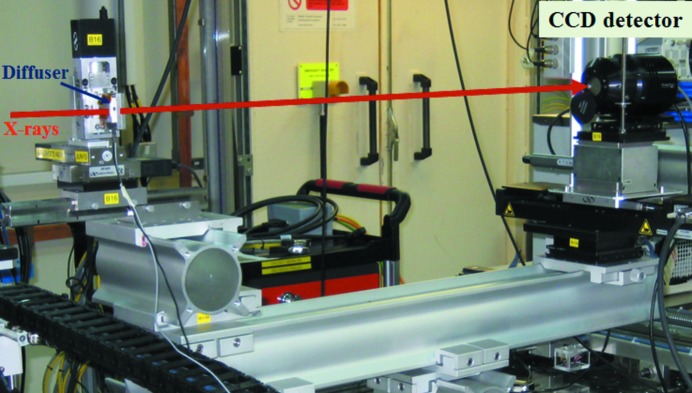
Photograph of the portable metrology device installed on B16 beamline for the characterization of X-ray mirrors. The mirror is installed upstream of the membrane stage and is therefore not visible in the above figure.
